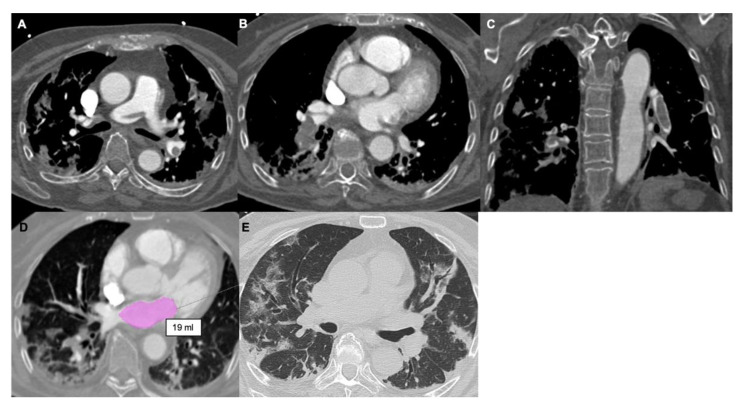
(A,B) Axial and coronal (C) contrast-enhanced CT scans show saddle embolism and multiple eccentric emboli involving the lower lobar branches of the pulmonary arteries. (D) Left atrium, purple. There is decreased LA volume, 19 mL. (E) Axial scans in the lung window show peripheral ground-glass opacities in association with consolidation areas involving both lungs.
